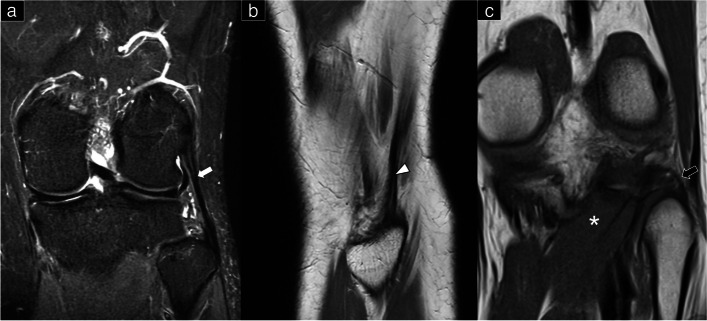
Magnetic resonance imaging shows the lateral collateral ligament in the coronal plane (a), biceps femoris tendon in the sagittal plane (b), and arcuate ligament in the coronal plane (c). White arrow: lateral collateral ligament; white arrowhead: biceps femoris tendon; black arrow: arcuate ligament; asterisk: popliteus
